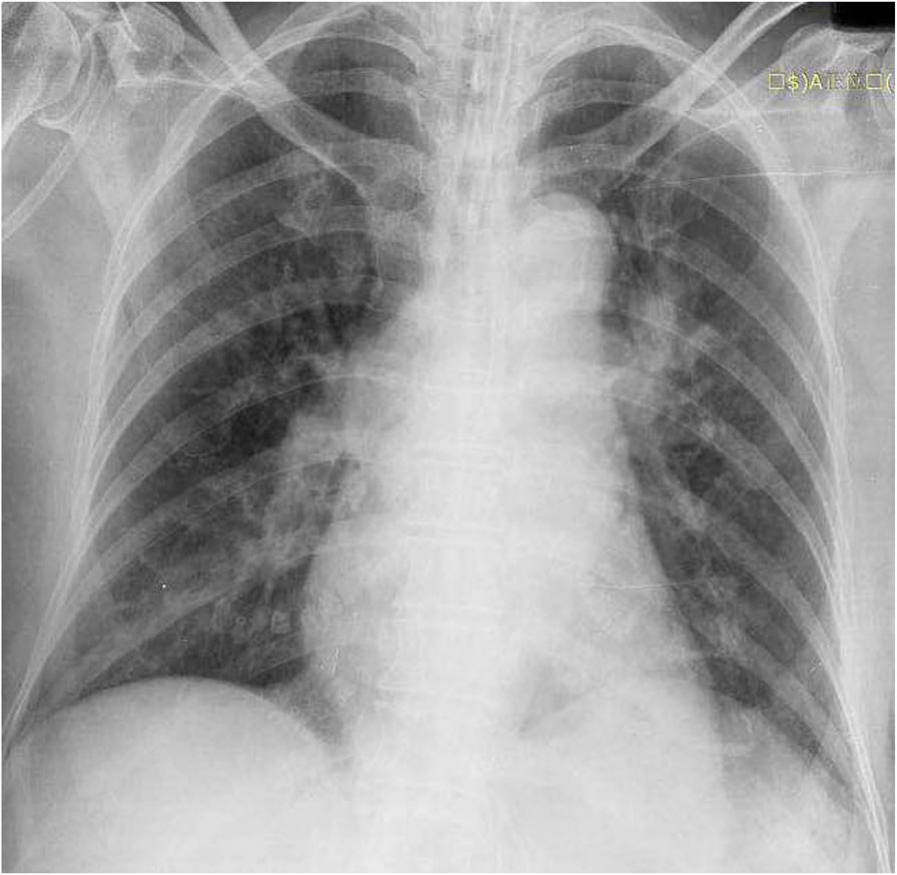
Chest x-ray demonstrating the pulse generator and the pacing leads have been removed completely
